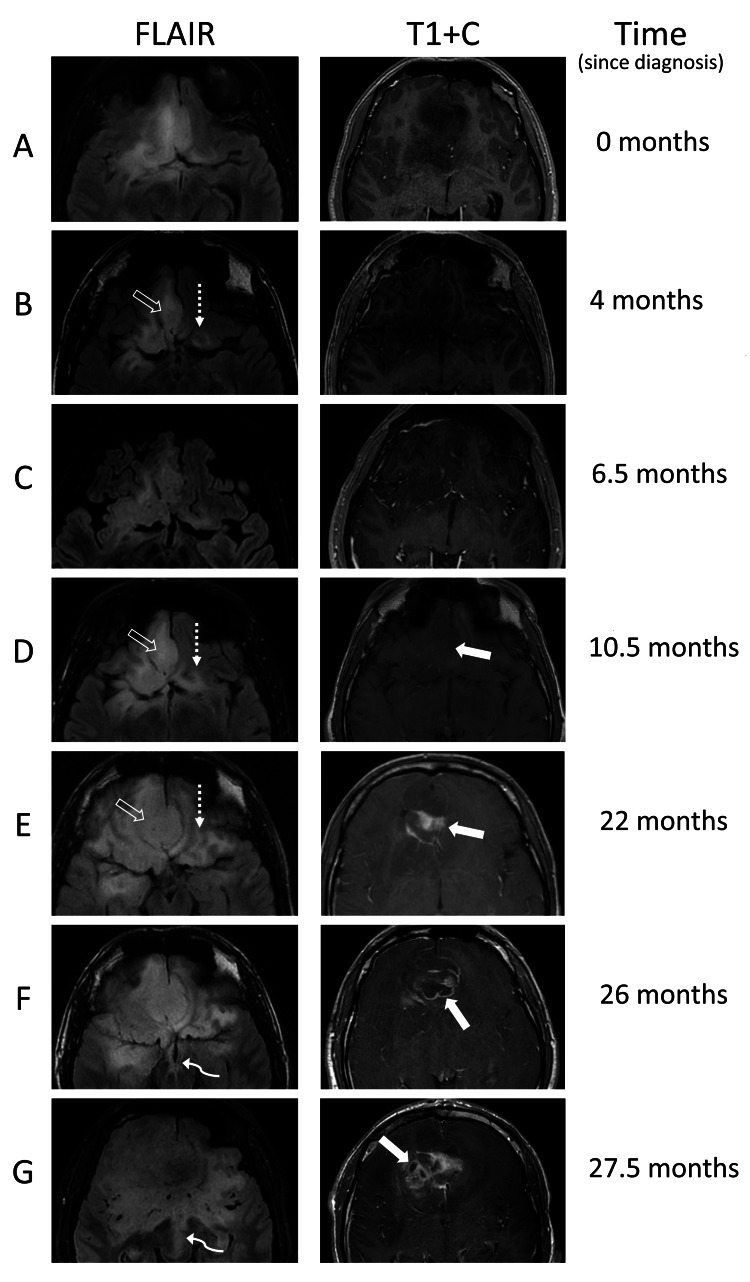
Axial MRI images showing subtle progression of gliomatosis throughout the brain over time A is the patient’s preoperative baseline scan demonstrating a nonenhancing, infiltrating glioma at the inferior aspect of the dominant frontal mass, into the bilateral inferior frontal lobes, right medial temporal lobe, and hypothalamus. B and C demonstrate no evidence of significant change in the immediate 2.5-month follow-up exam, however, disease progression can be better seen over longer periods of time, such as open arrows in B, D, and E, showing increased fullness and mass effect in the right gyrus rectus. Dashed arrows in B, D, and E show the same phenomenon of progressive gliomatosis in the left orbitofrontal gyri. Curved arrows in F and G show the progression of gliomatosis into the midbrain. Solid arrows in D and E demonstrate interval enhancement of the tumor, indicating progression into a higher-grade tumor, with the eventual development of necrotic areas as evidenced by the solid arrows in F and G. FLAIR: fluid-attenuated inversion recovery
